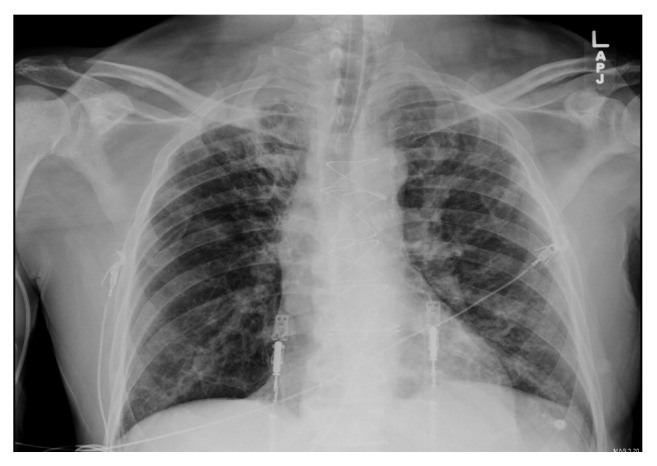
Preoperative chest X-Ray (CXR).
